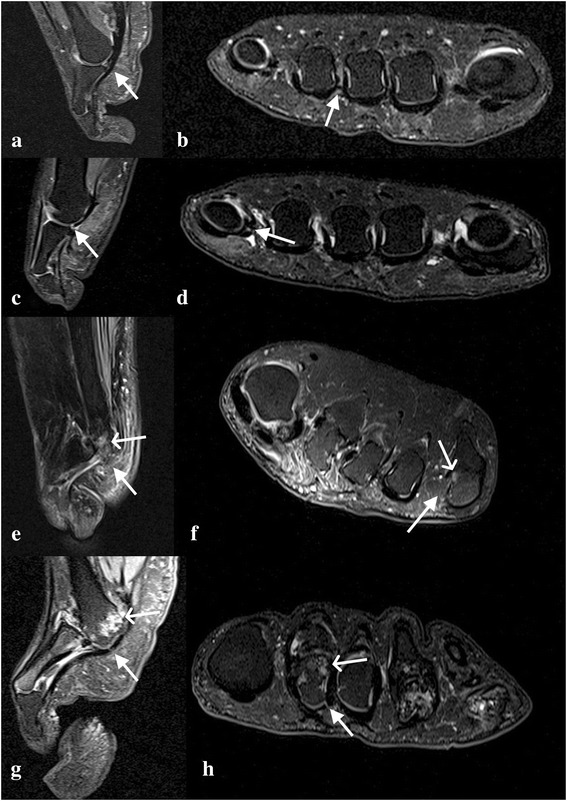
Intermediate-weighted, fat-suppressed sagittal (a) and short axis (b) MR images of 3rd MTP joint demonstrating an intact capsule and plantar plate (white arrow) in a control subject. Sagittal (c) and short axis (d) images of a central-distal plantar plate tear (white arrow) at the 5th MTP joint in a control subject. Sagittal (e) and short axis (f) images demonstrating an absent plantar plate (closed arrow) and erosion (open arrow) at the 5th MTP joint in a patient with RA. Sagittal (g) and short axis (h) images demonstrating a tear (high signal) (closed arrow) in the plantar plate and erosion (open arrow) of the 2nd MTP joint in a patient with RA
